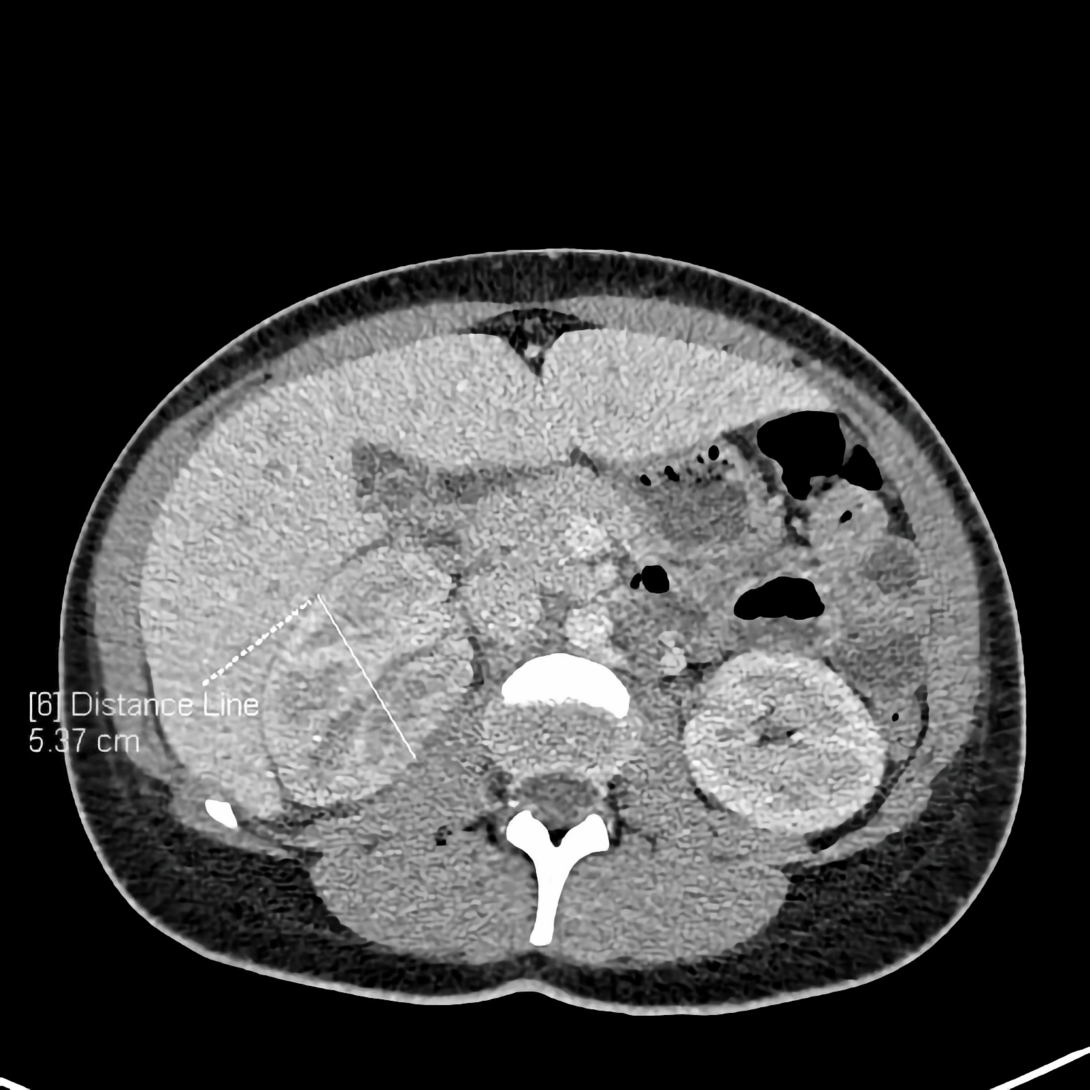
CT scan (axial) showing enlarged right kidney CT: computed tomography
